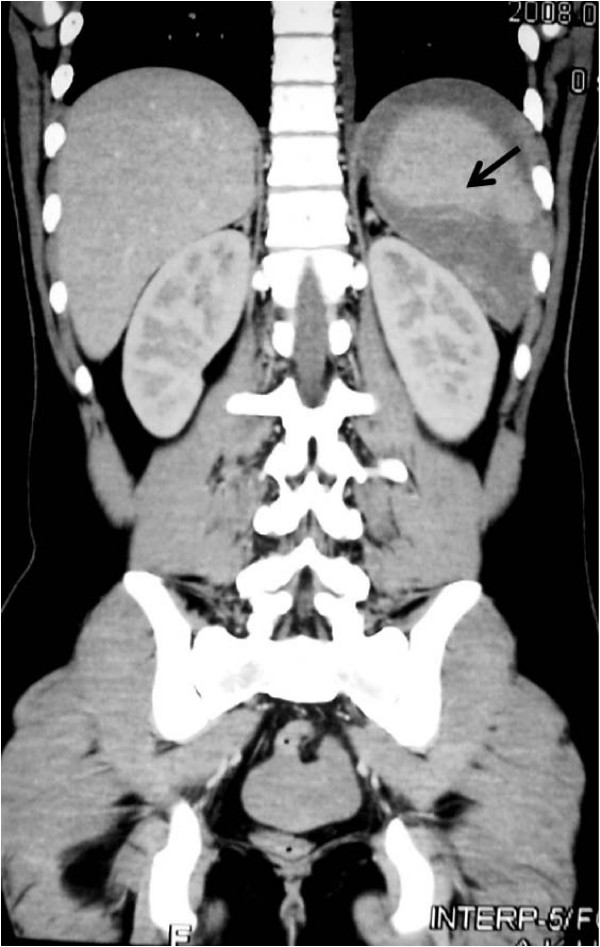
CT SCAN Axial cuts showing peri-splenic fluid collection (bleed) and Niche of rupture (arrow).
